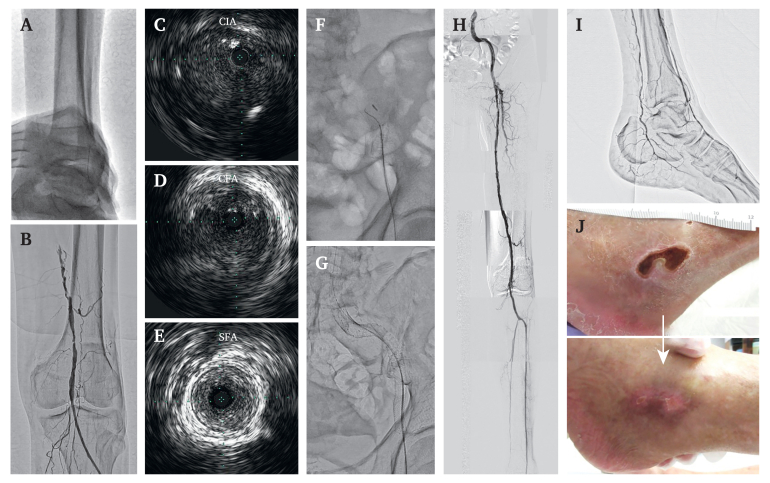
Transankle intervention for long segment occlusive disease on the left side. (A) A guidewire was inserted after puncturing the dorsalis pedis artery with extravascular ultrasound guidance. (B) Initial angiography showing the exit of the superficial femoral artery occlusion, representing intravascular ultrasound short axis images of the guidewire passing route. (C) Common iliac artery. (D) Common femoral artery. (E) Superficial femoral artery. (F) Repeated parallel wiring to trace the intraplaque route. (G) Deployment of three SMART stents from the common iliac artery ostium to the external iliac artery. (H) Final angiogram confirming vascular patency and favourable blood flow in the left lower extremity. (I) Confirmation of the intact left dorsalis pedis artery 19 days after the second intervention. (J) Wound healing in the left lower limb 35 days after the second intervention.
